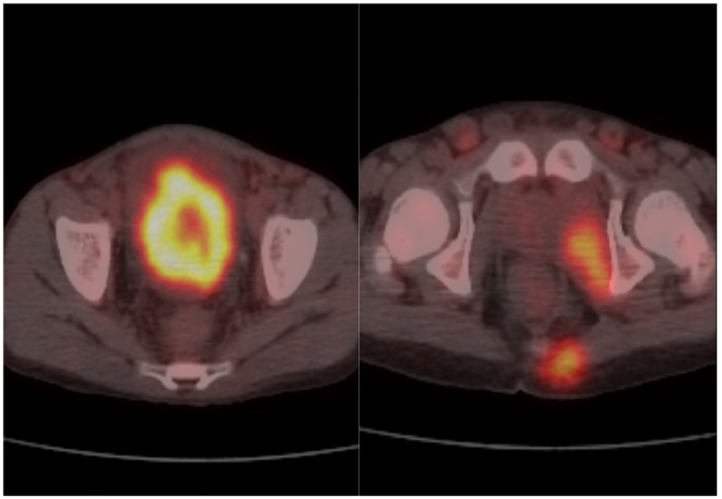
Axial 18F-FDG PET CT scan fused image showed (A) hypermetabolic pelvic mass, and (B) hypermetabolic left internal obturator muscle, partially visualized left gluteal lesion and mildly hypermetabolic bilateral inguinal lymph nodes.
